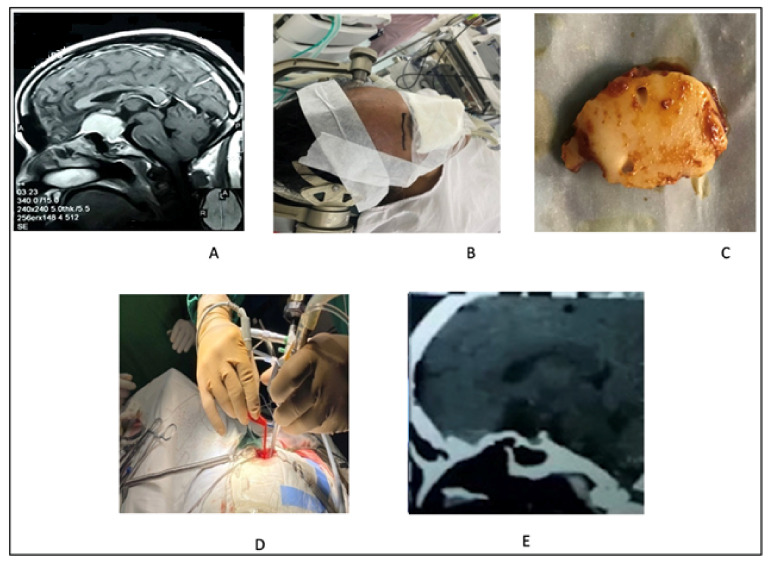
(A) Sagittal view MRI with contrast shows medium-sized TSM. (B) Planning for superciliary keyhole endoscopic approach. (C) Small piece of frontal bone in keyhole approach. (D) Picture shows three hand technique in superciliary keyhole endoscopic-assisted approach. (E) Post-operative CT scan in sagittal view showing no residual tumor.
